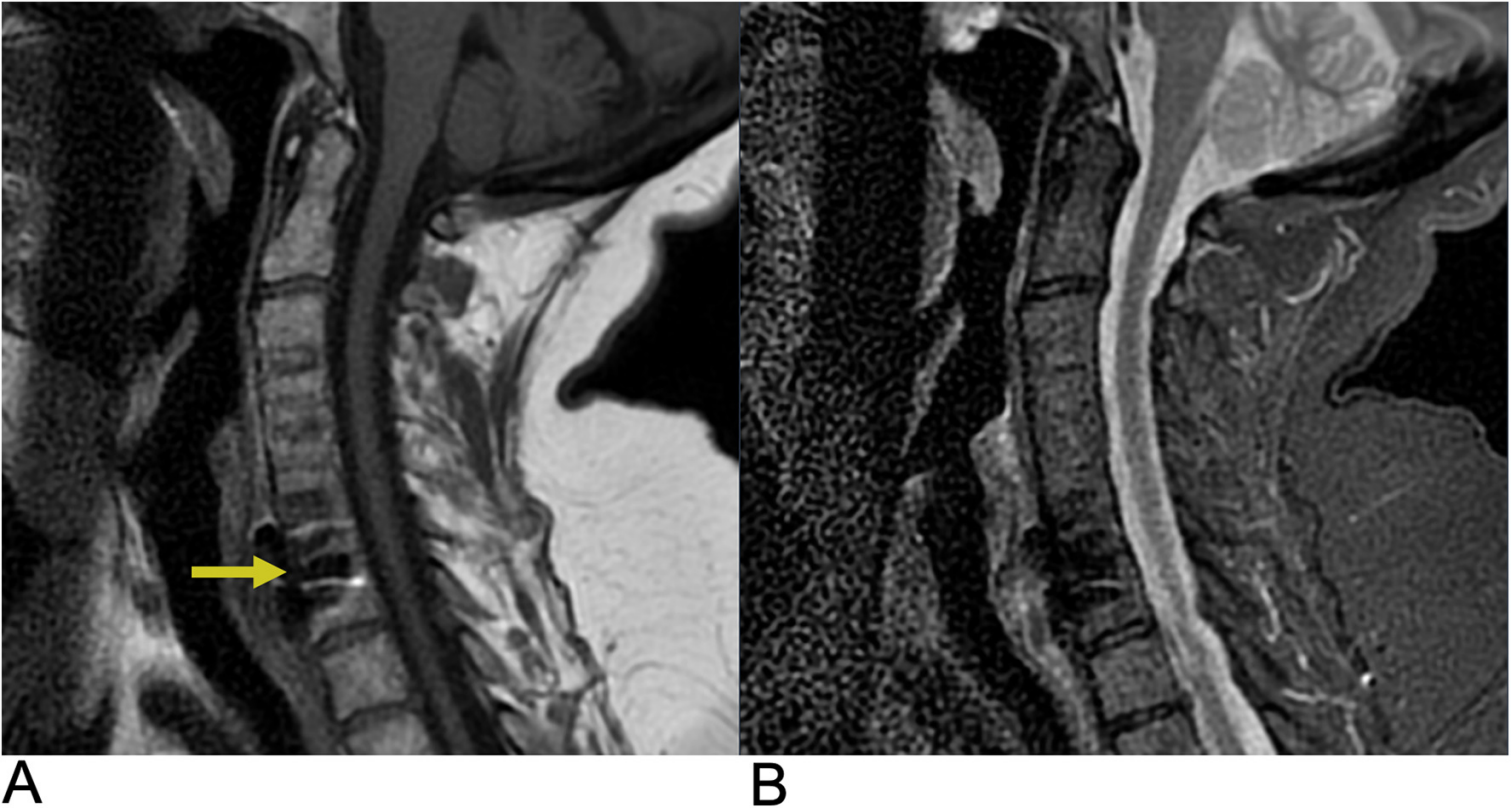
Block vertebra. Sagittal T1 (A) and sagittal T2 (B) MRI demonstrate absent intervertebral disc space due to congenital block vertebra resulting from failed vertebral segmentation. There is T1 hypointensity (yellow arrow) and T2 hyperintensity in adjacent soft tissues suggesting secondary changes or abnormal spinal curvature.
